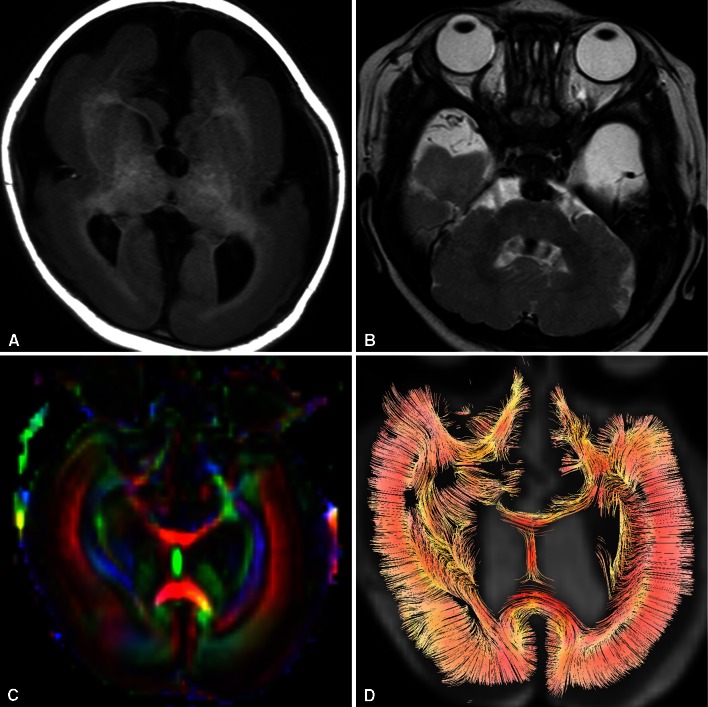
T1-weighted axial image (a) and T2-weighted axial images (b) show diffuse thickening of the cortex with lack of sulcation, accompanied by severe dysplasia of the brainstem and cerebellum. Obscuration of the contour of the basal ganglia and internal capsule is also demonstrated. FA colormap (c) and tractography (d) reveal radially oriented anisotropic structure within the thickened cortex, which is not normally observed after birth
